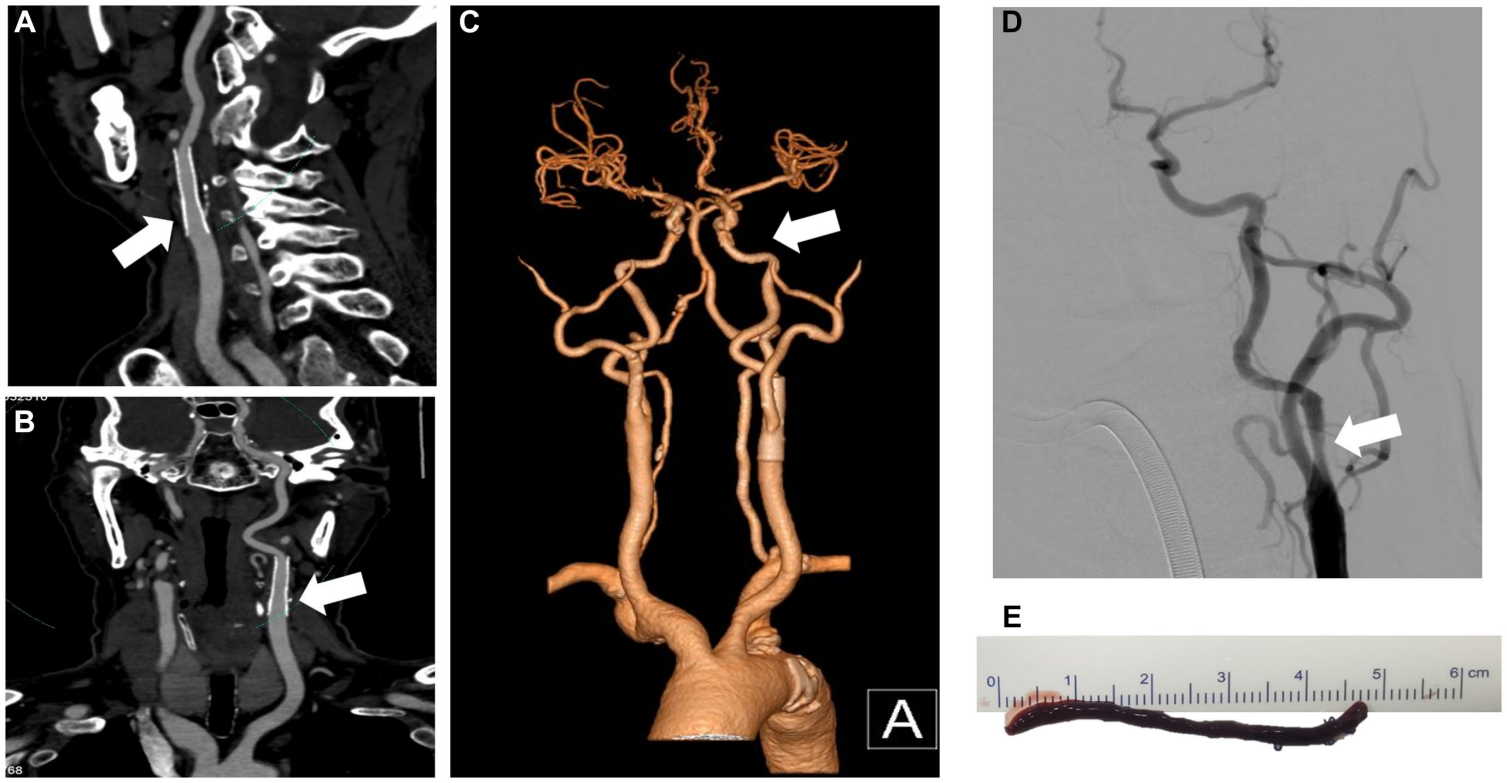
CTA and DSA show no residual stenosis and no thrombosis after mechanical thrombectomy. The sagittal plane (A), coronal plane (B), and 3D reconstruction (C) of the CTA show carotid artery recanalization. DSA shows no signs of thrombosis (D). The 4.6-cm red blood clot was removed from the left internal carotid artery after mechanical thrombectomy (E). CTA, computed tomography angiography; DSA, digital subtraction angiography; 3D, three-dimensional.
